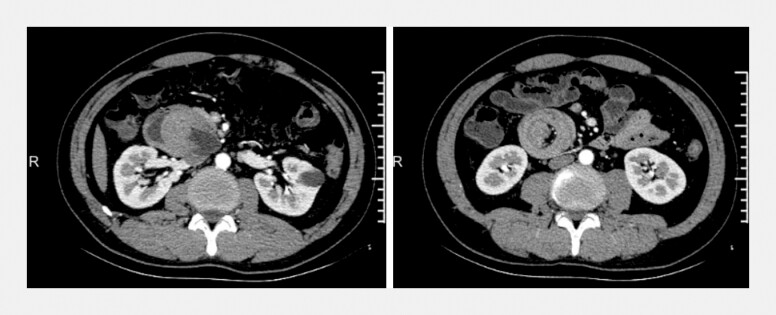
Contrast-enhanced abdominal CT showing a nodular soft tissue density in the descending duodenum, with partial jejunal intussusception into the duodenal lumen. CT, computed tomography.
